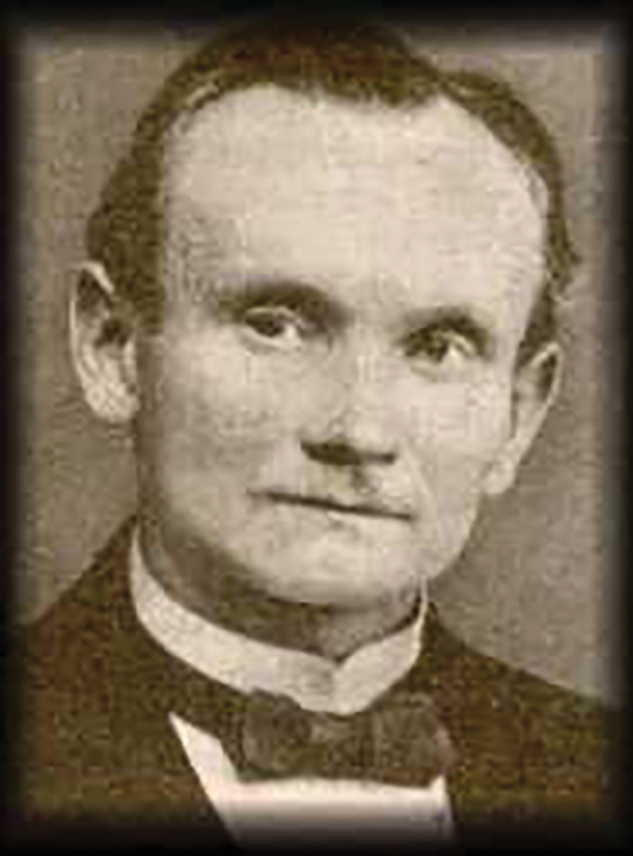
Reinhold Burger (1866–1954). In early 1896, he was a pioneer in manufacturing X-ray tubes in Berlin [47].
